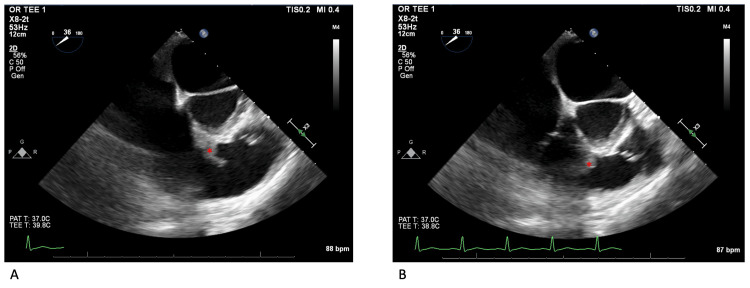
Transesophageal Echocardiography Figures 2A-B depict the TEE midesophageal RV inflow/outflow view. Figure 2A shows the vegetation extended towards the RV outflow tract in the septal aspect of the TV subvalvular apparatus. Figure 2B displays further extension of the vegetation into the RV outflow tract, which was concerning for a dynamic partial RV outflow tract obstruction. The red asterisk marks indicate the infectious vegetations. TEE: Transesophageal echocardiogram; RV: Right ventricle; TV: Tricuspid valve
